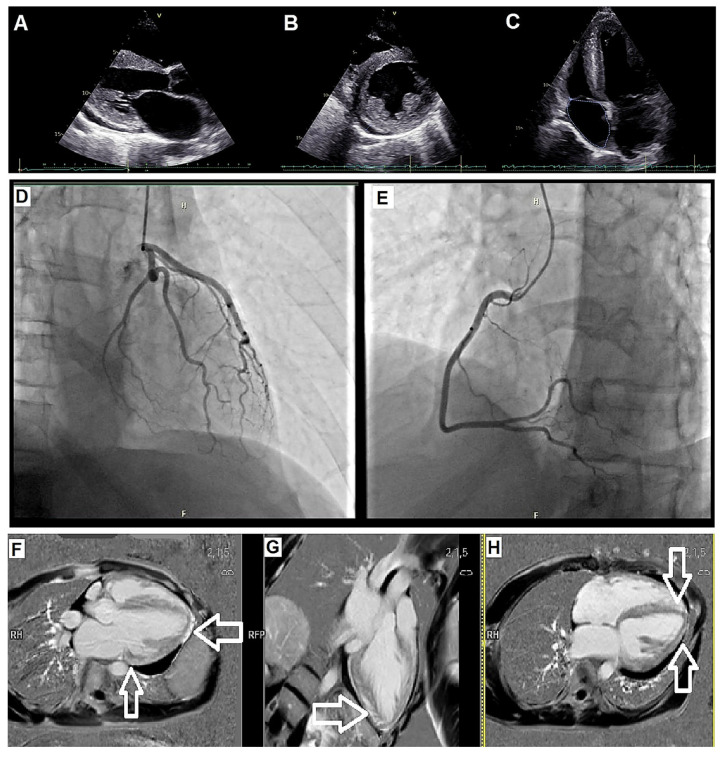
Echocardiography: (A) Left ventricular (LV) hypertrophy from Parasternal Long Axis (PLAX) with pericardial effusion behind the lateral wall. (B) LV hypertrophy from Parasternal Short Axis (PSA) with pericardial effusion. (C) LV hypertrophy from Apical 4 chamber (A4C). Negative selective coronary angiography: (D) Basin of the right coronary artery. (E) Basin of the left coronary artery. Cardiac Magnetic Resonance: (F) Arrows indicate diffuse left ventricular hypertrophy (LVH), mainly anteroseptal and lateral. Late gadolinium enhancement (LGE) shows replacement fibrosis, suggesting myocardial remodeling. (G) Arrow highlights hypertrophied myocardium in the basal anteroseptal and inferolateral segments. LGE confirms myocardial fibrosis with transmural involvement. (H) Arrows mark fibrotic areas in the basal and midventricular regions of the left ventricle. Transmural LGE suggests advanced myocardial damage and remodeling.
